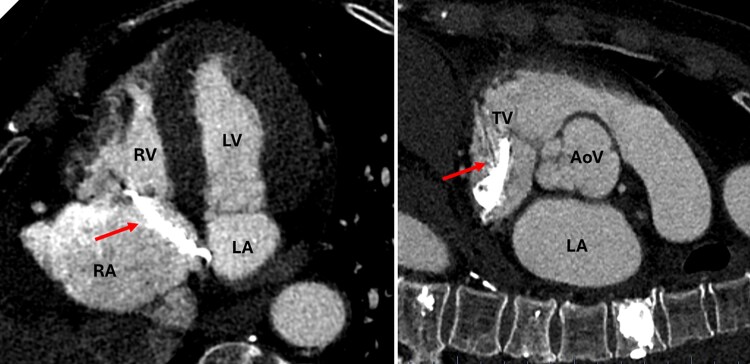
CT angiography multiplanar reconstructions showing the cement mass and its relation with cardiac structures. During post-processing, the projections were reformatted to match standard echocardiographic imaging planes, facilitating comparison and precise assessment of cardiac structures. AoV, aortic valve; LA, left atrium; LV, left ventricle; RA, right atrium; RV, right ventricle; TV, tricuspid valve.
